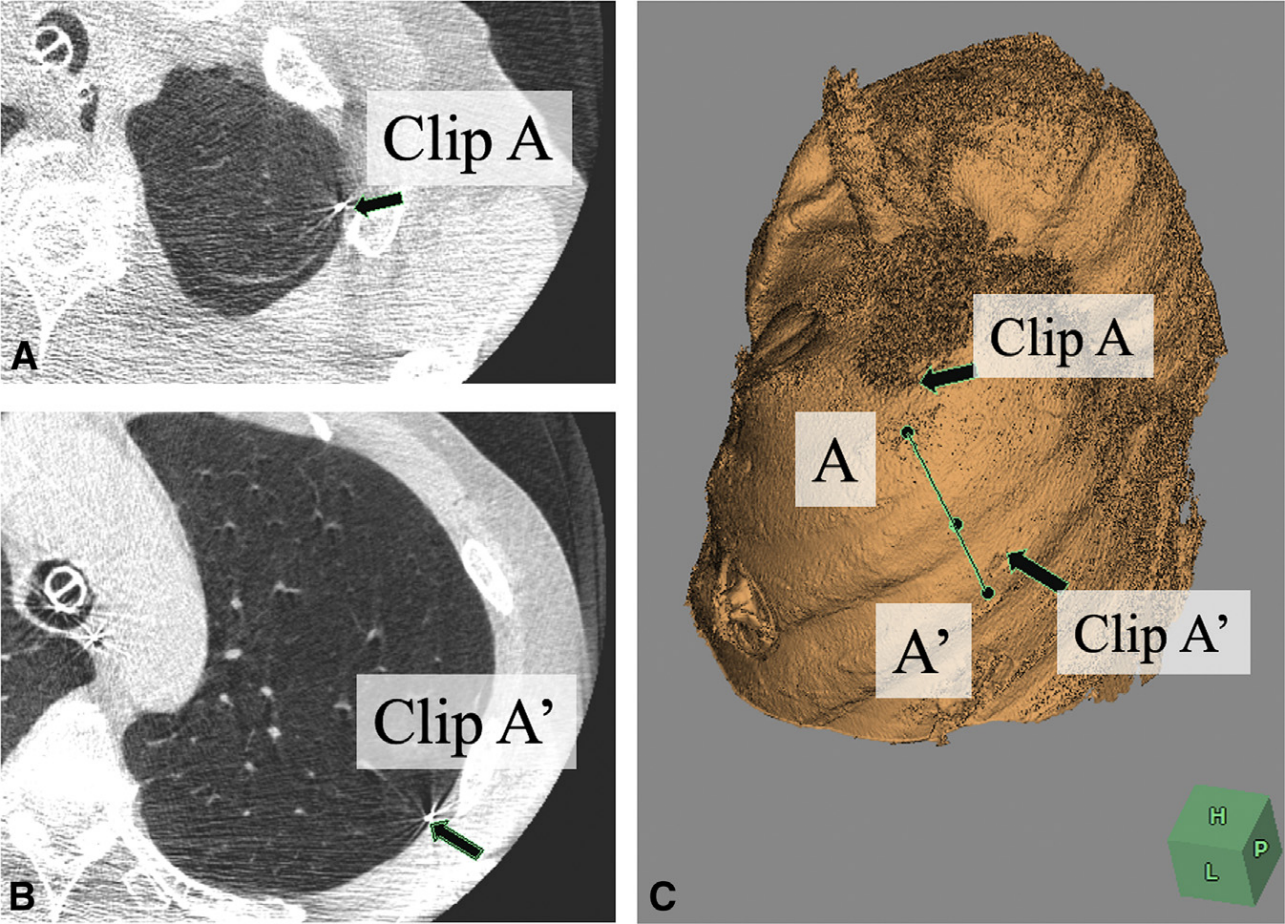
Intraoperative cone-beam computed tomography obtained after clipping of approximated points A and A' under bilateral ventilation (A and B). The correct points A and A' were determined on the basis of the multiplanar reconstruction images on cone-beam computed tomography, and the discrepancies between the clipped point A and the correct point A, as well as the clipped point A' and the correct point A' were measured (C).
